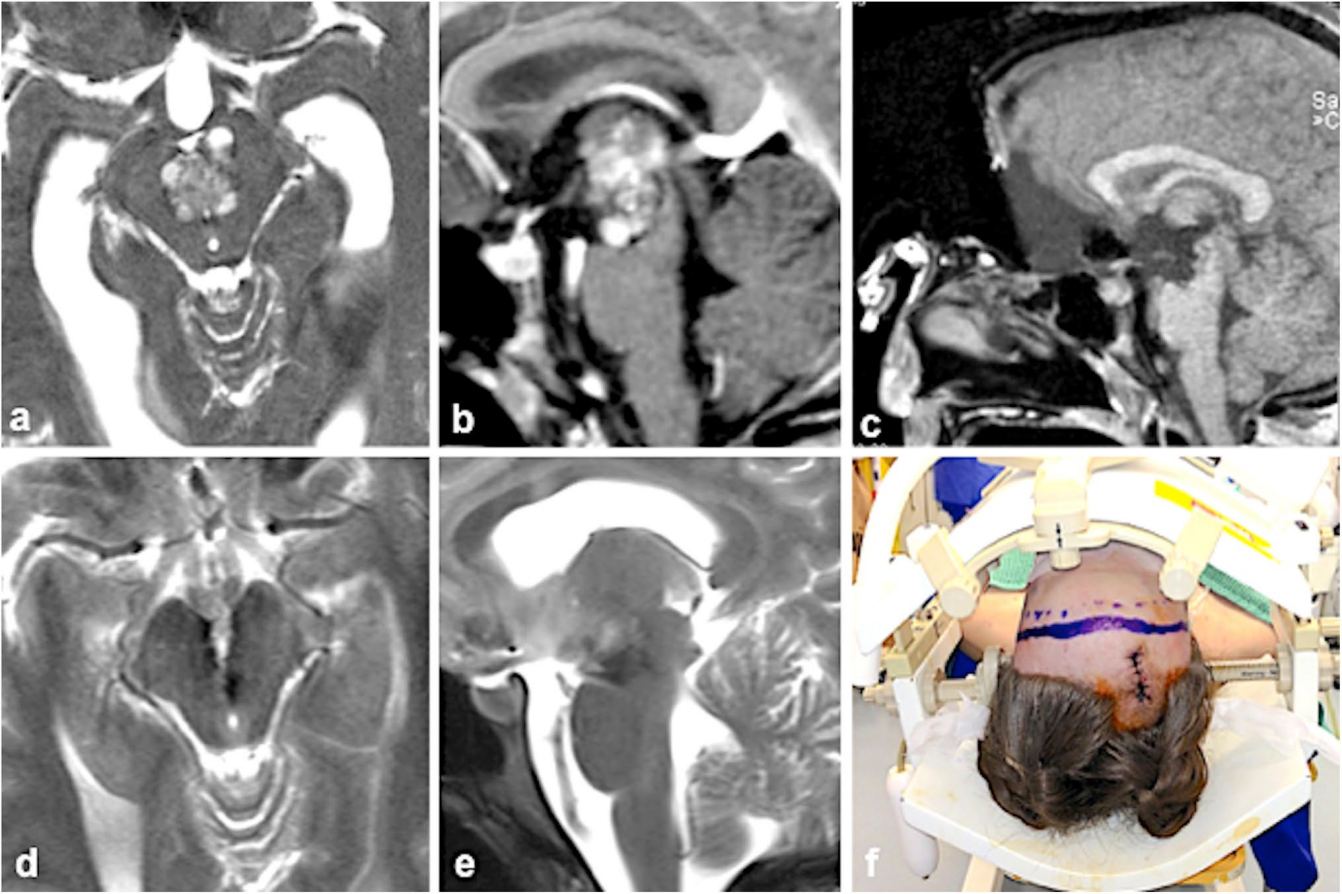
This 21-year-old woman presented with transient oculomotor nerve palsy; the MRI revealed a centrally located MCM (a) with thalamic extension whose upper part reached the floor of the third ventricle (b). Due to diplopia, severe headache, and vomiting caused by obstructive hydrocephalus, she underwent endoscopic third-ventricle ventriculostomy. Several days later, surgical MCM resection was undertaken via the anterior interhemispheric approach; the sagittal intraoperative MRI demonstrates complete lesionectomy (c); axial (d) and sagittal post-surgical MRI also confirmed complete MCM resection (e). The head was fixed in a special headrest with MRI coils; a coronal incision is marked on the skin (f). Postoperatively, slightly accentuated oculomotor nerve palsy was present that normalized within 3 months
